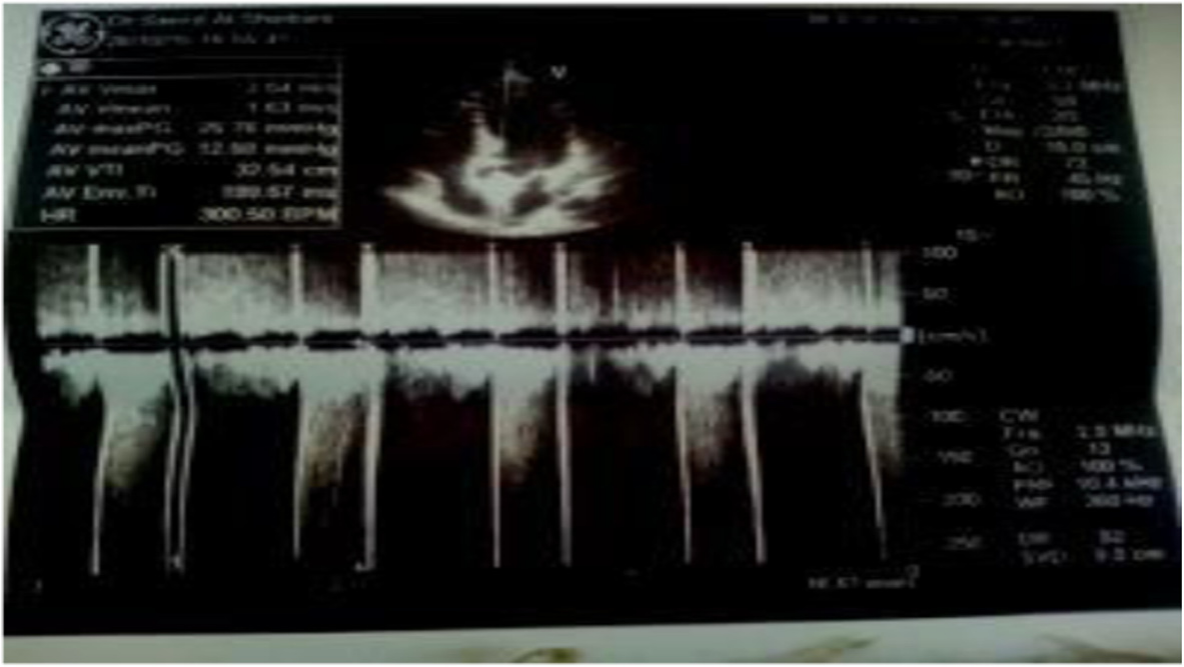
Transthoracic echocardiography of patient’s mechanical prosthetic aortic valve; mean gradient was 12.58 mmHg
